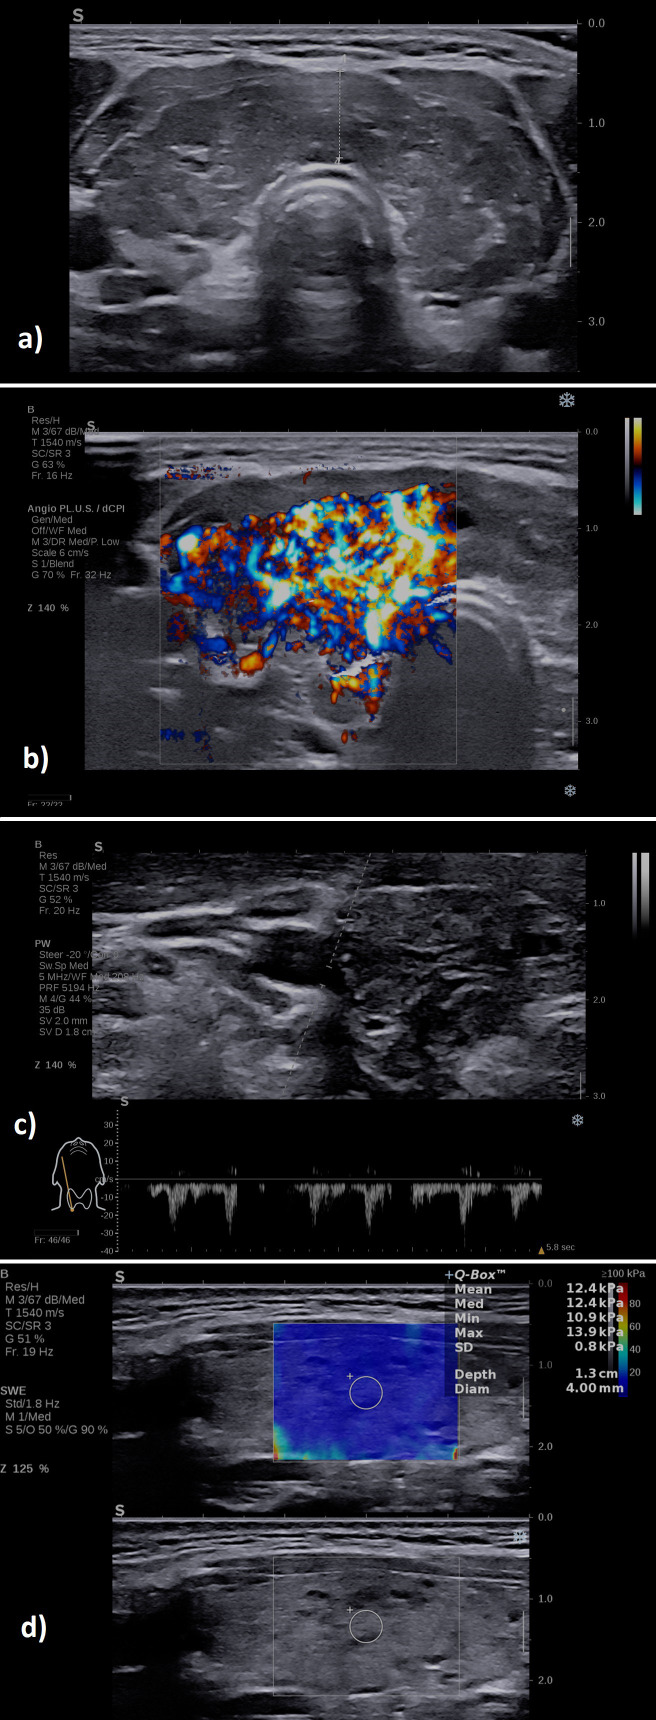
A case of a patient with subacute thyroiditis: (A) B-mode examination of the right thyroid lobe in sagittal plane: diffusely hypoechoic; (B) with increased vascularity grade II Color Doppler, image is obtained using the fine-flow mode called ANGIOPLUS on the equipment used in this study; (C) spectral Doppler lower PSV in the thyroid artery; (D) increased mean elasticity index measured in 2D SWE 109.7 kPa.
